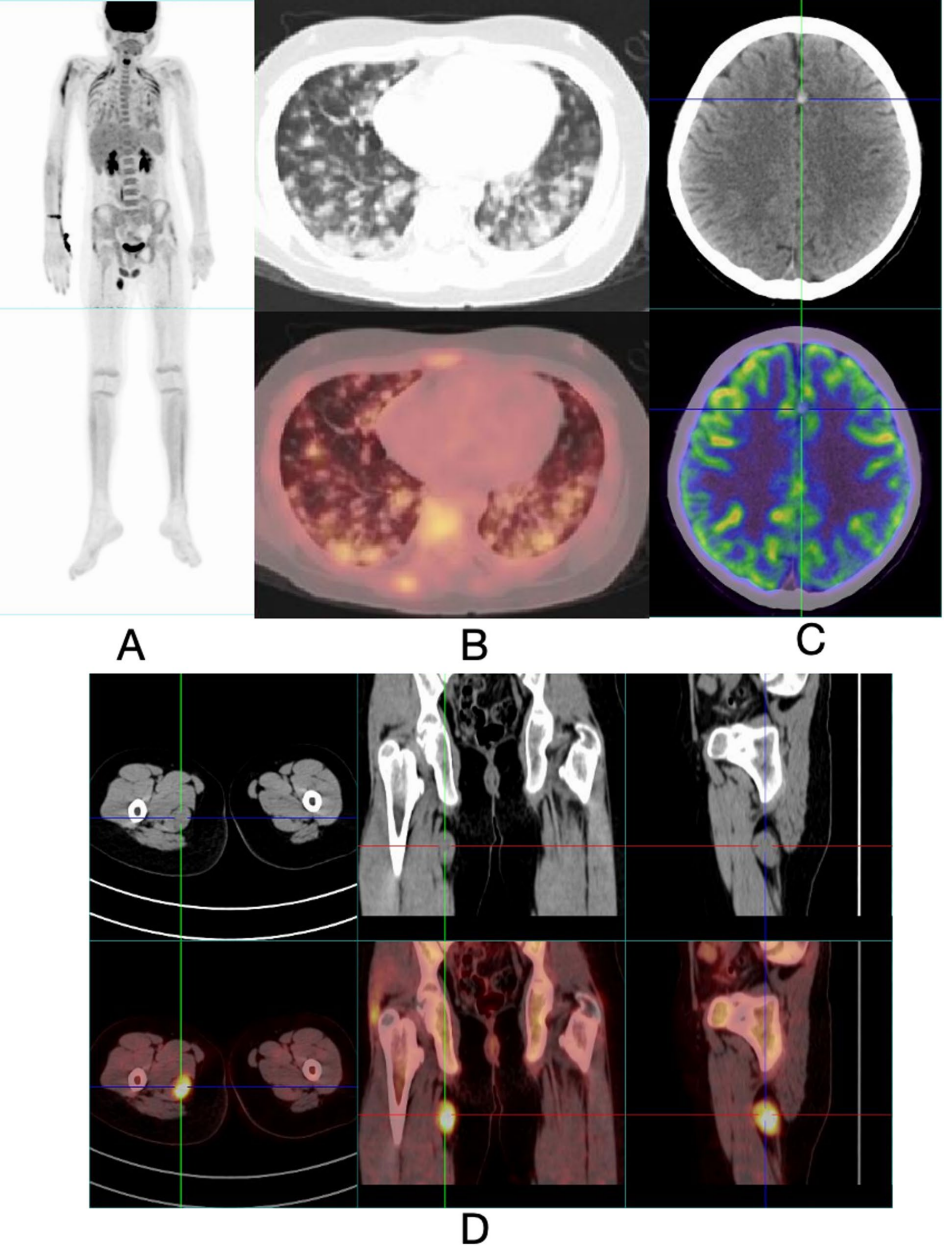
A: Maximum intensity projection (MIP) image of whole-body PET-CT. B: PET-CT of the lungs demonstrated diffuse, multifocal, small central nodules with moderate FDG uptake (SUVmax: 2.7). The lesions were more prominent in the bilateral lower lobes, accompanied by adjacent pleural thickening and traction, with increased FDG uptake (SUVmax: 1.5). C: PET-CT of the brain revealed a cortical nodule in the temporal lobe without abnormal FDG uptake. D: PET-CT identified a soft tissue mass (size: 2.6 cm × 1.7 cm × 3.0 cm) in the right thigh with significantly increased FDG uptake (SUVmax: 8.8)
